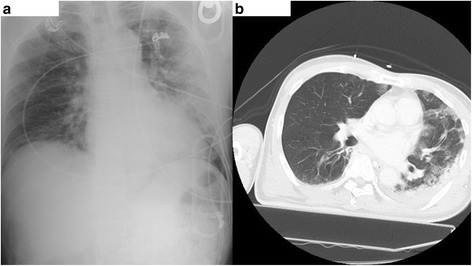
Imaging studies on admission. a Chest X-ray. *Left* dominant bilateral infiltration shadow is noted. b Computed tomography scan. *Left* dominant bilateral infiltration shadow with *small* pleural effusion is noted
